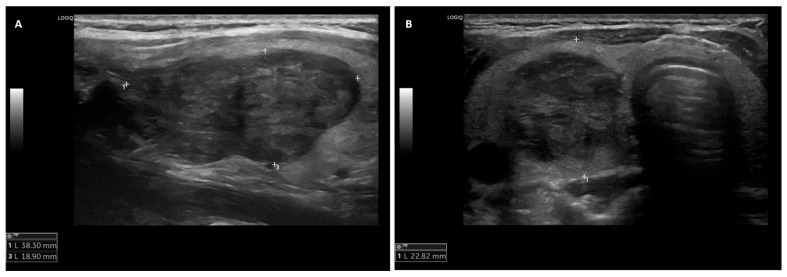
Ultrasound imaging of the thyroid right lobe. (A) Nodule longitudinal scan and (B) nodule axial scan.
